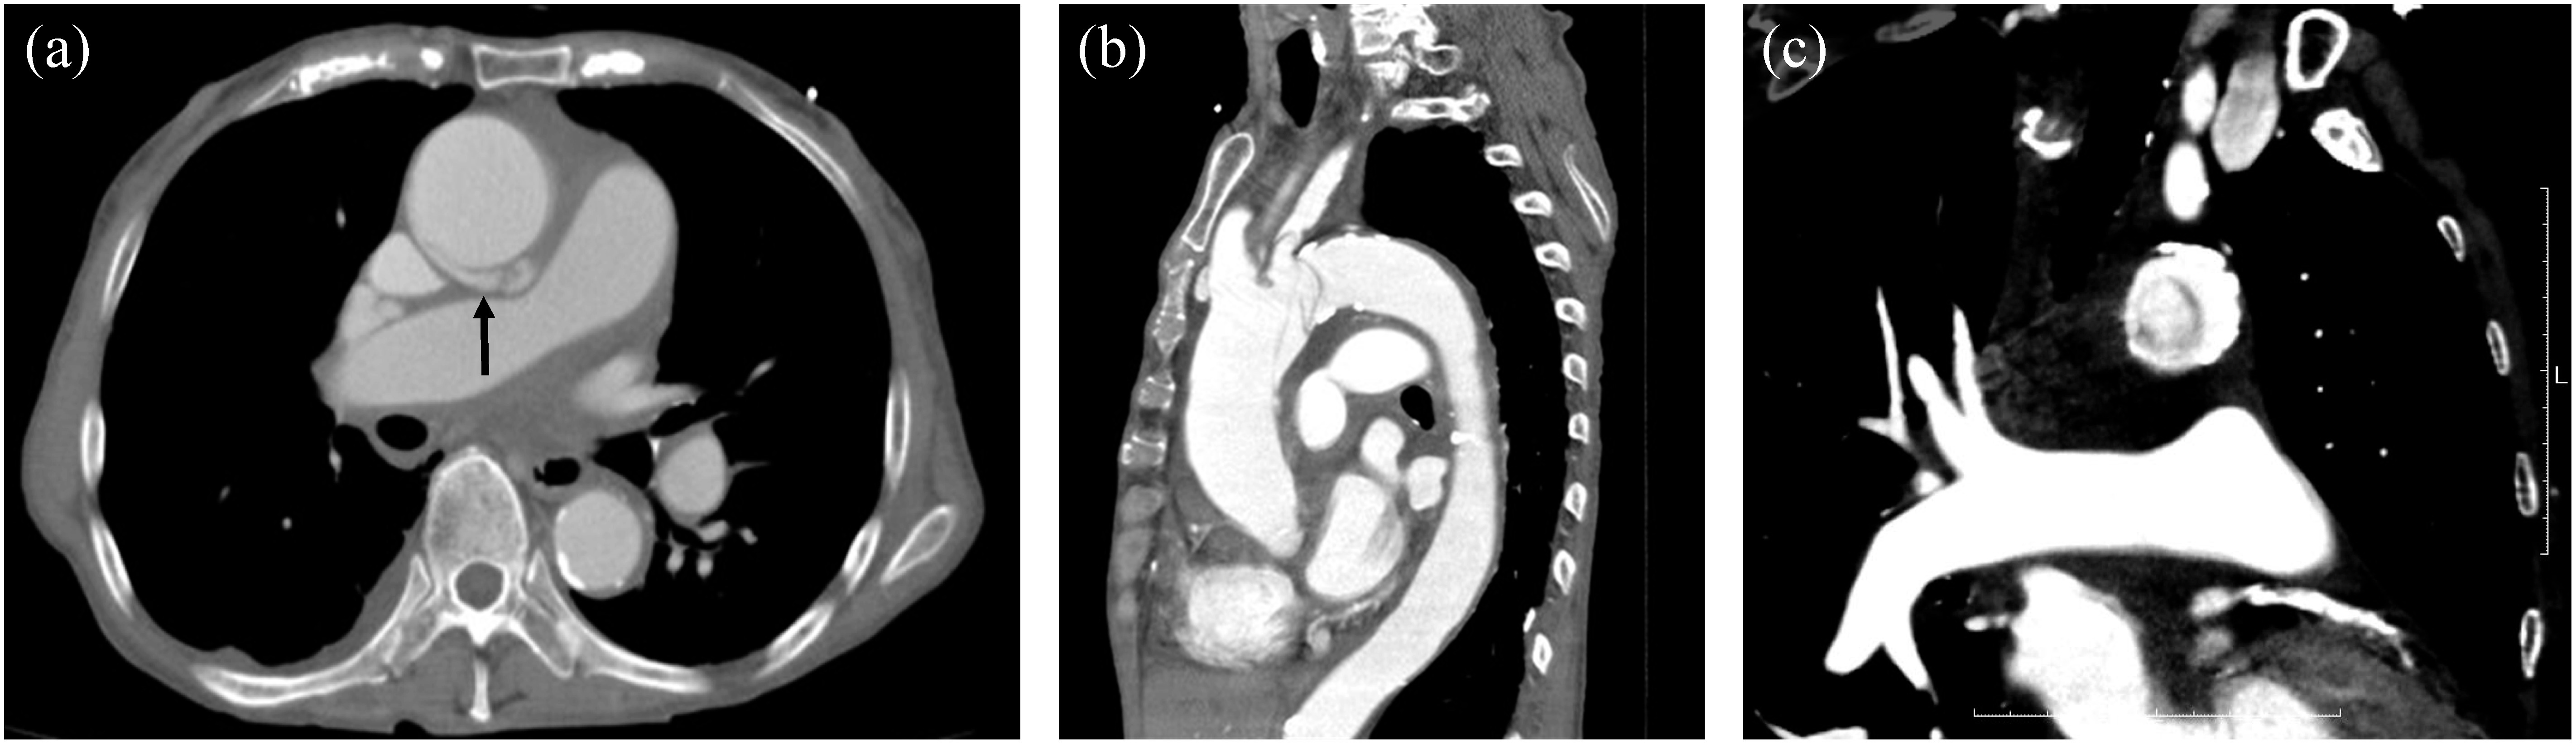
Fig. 1 Contrast-enhanced computed tomography. (a)–(c) show axial, sagittal, and oblique views, respectively. In (a) and (b), the intimal flap cannot be found in the mid-ascending aorta. (a) An extravasation of contrast medium to the posterior wall of the proximal ascending aorta (arrow). (b) An intimal flap is seen from the distal ascending aorta to the aortic arch. (c) An intimal flap is seen as concentric circle at the aortic arch.
